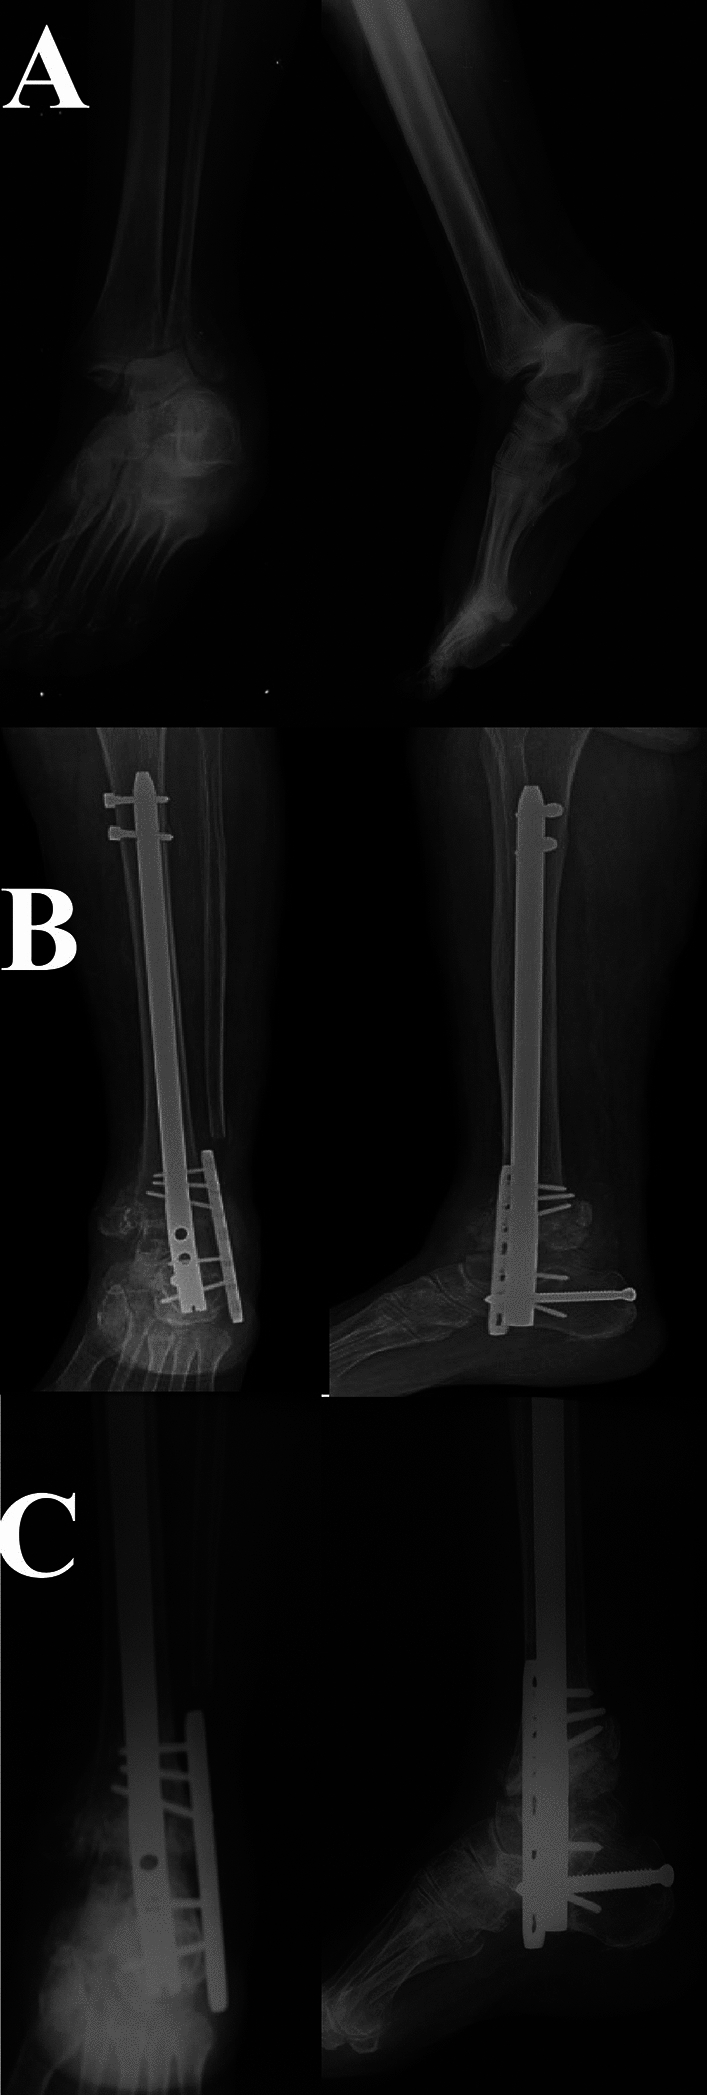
A female patient 55 years old presented with ankle fracture dislocation (OTA/AO 44B3) (HbA1C: 7.5 and AFDA score 7). A Preoperative radiographs. B Immediate postoperative radiographs. C At 10 months, follow-up radiographs showed stable implants and ankle arthrodesis
